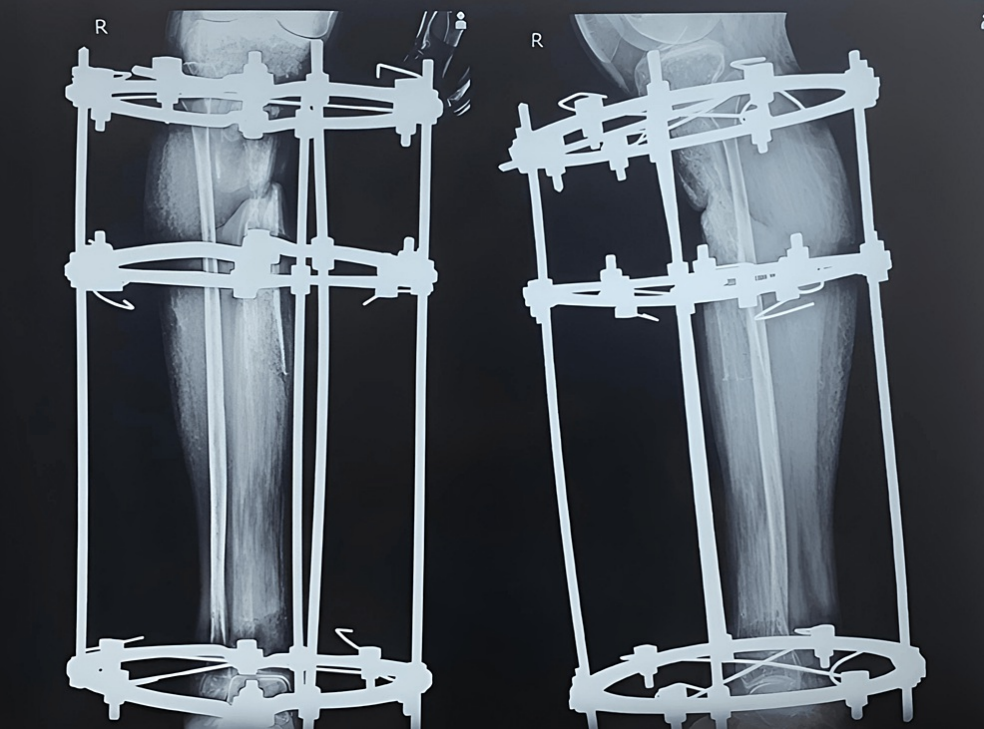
X-ray of follow-up on March 2021.
